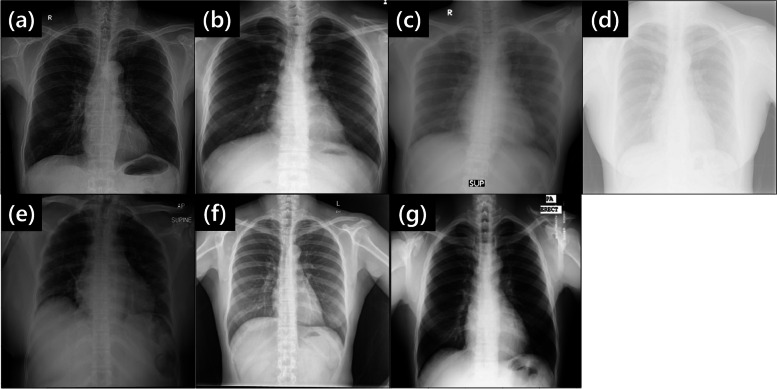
Example chest x-ray images of different x-ray machine specifications: (a) VH_DR1_, (b) IH_DR2_, (c) IH_CR1_, (d) IH_CR2_, (e) IH_CR3,Mobile_, (f) SZ_DR3_, and (g) MG_CR4_. Each image reveals unique characteristics (*e.g.*, contrast and noise levels). The images were plotted without adjusting the window level and width except for SZ_DR3_ and MG_CR4_. In other words, raw image data were extracted from DICOM files
